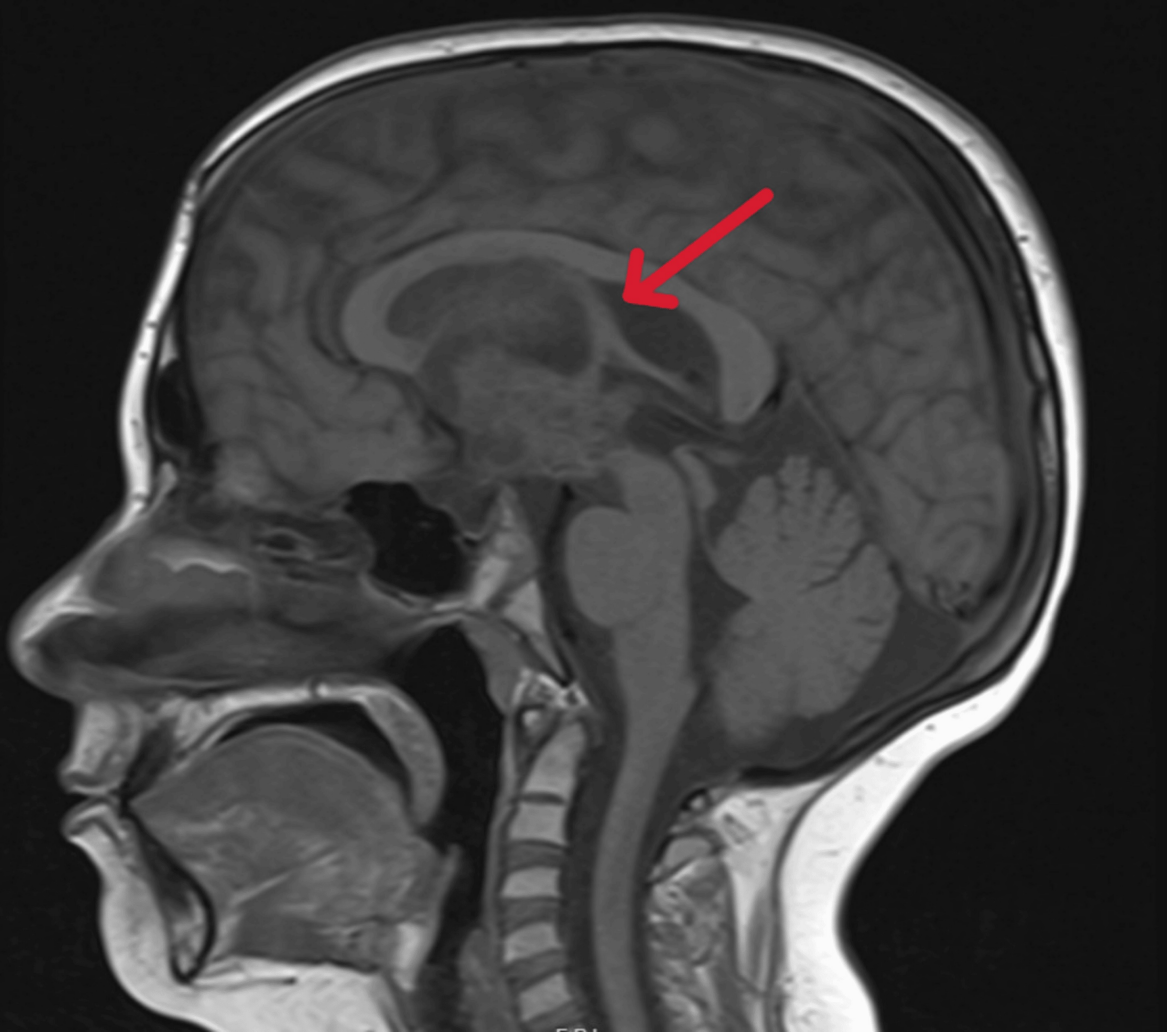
MRI brain with and without contrast, 2024 The arrow depicts craniopharyngioma with enlarged solid and cystic components. Heterogenous solid component of the mass extended more posteriorly involving hypothalamus region protruding into the third ventricle and extending towards the interpeduncular cistern. Dorsally it extended and completely obstructed the foramen of Monro. The solid component of the mass approximately measured 3.2 x 2.5 cm.
